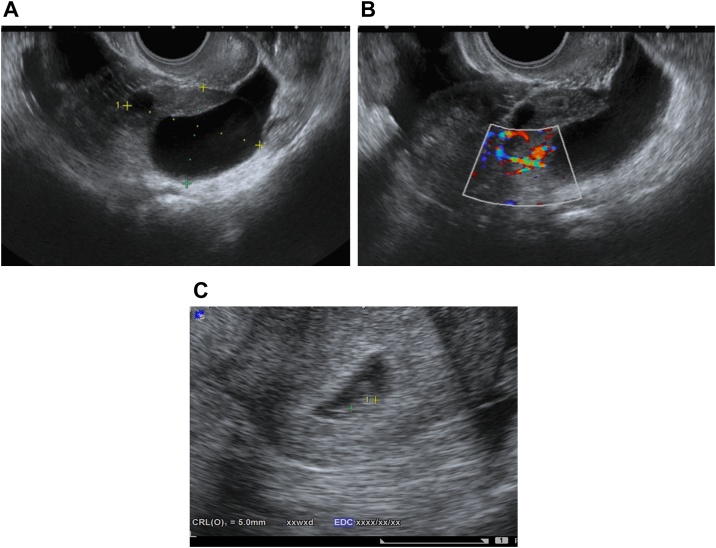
(A–C) Transvaginal ultrasound examination in the 6th week of gestation revealed a viable, singleton intrauterine pregnancy. The crown-rump length was 5.0 mm, and the patient had bilateral enlarged ovaries (right: 6.9 cm × 4.5 cm, left: 4.5 cm × 3.2 cm) with scanty ascites. Doppler ultrasonography revealed normal ovarian blood flow.
